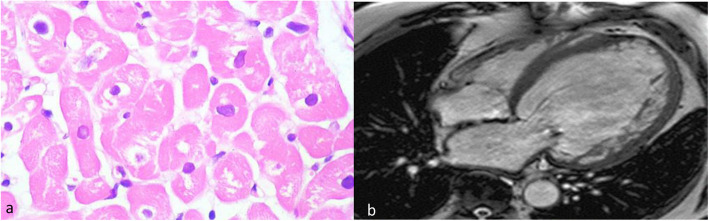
a Endomyocardial biopsy of the proband showing hypertrophy, variable nuclear enlargement with sarcoplasmic vacuolar degeneration of the myocytes, and absence of inflammation. b Cardiac magnetic resonance imaging of the proband with ejection fraction 14% and dilated thinning out of the left ventricle
